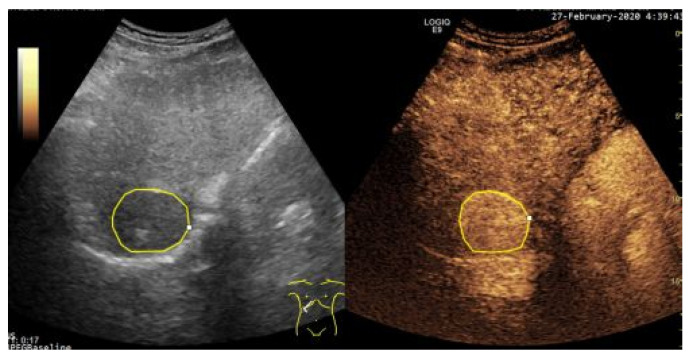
Example of a Hepatocellular Carcinoma (HCC) tumor within B-mode Ultrasound (left) and Contrast-Enhanced Ultrasound (CEUS) images (right), delineated by an experienced radiologist.
